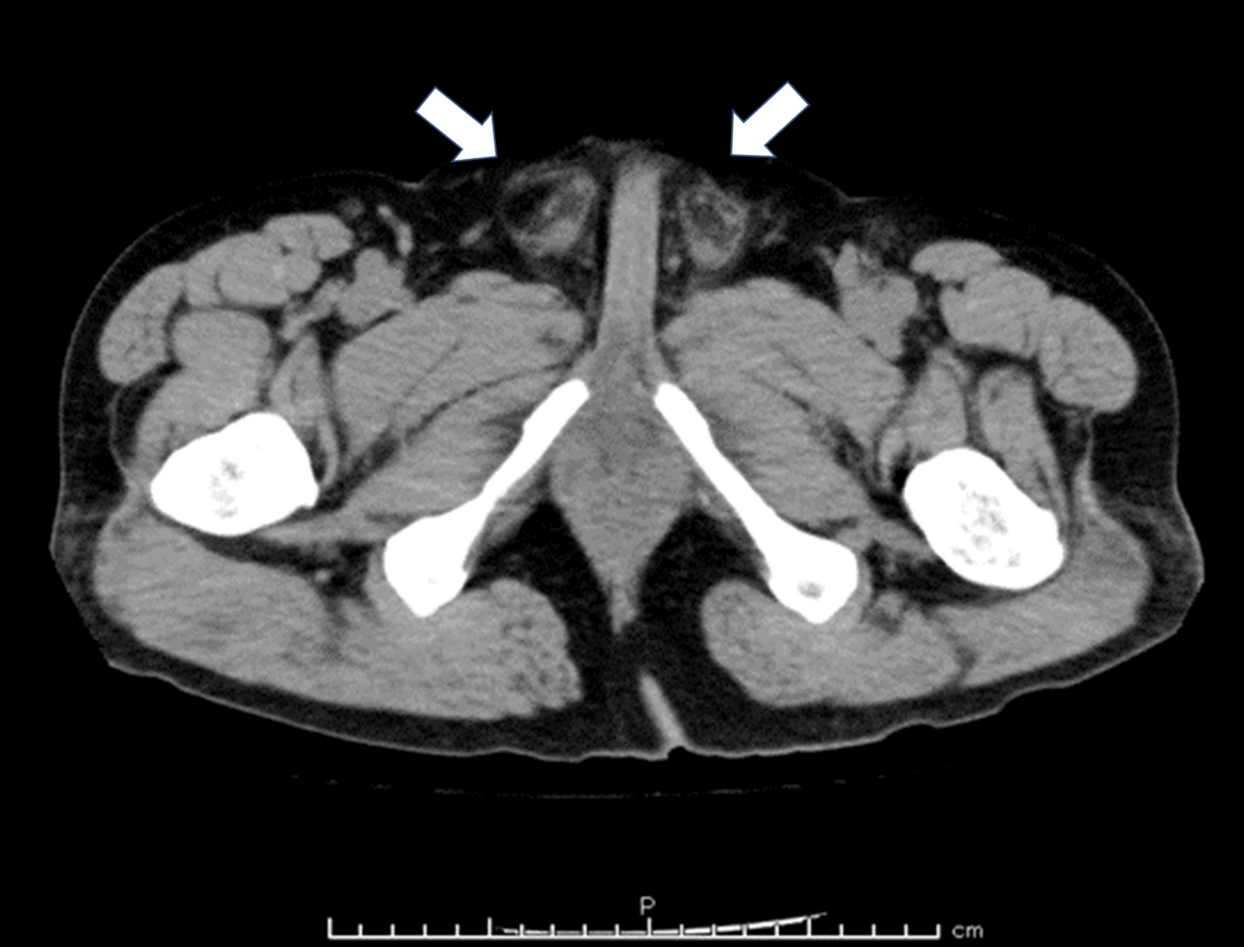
Pelvic CT Increased fat density was observed in both testes (white arrows).
